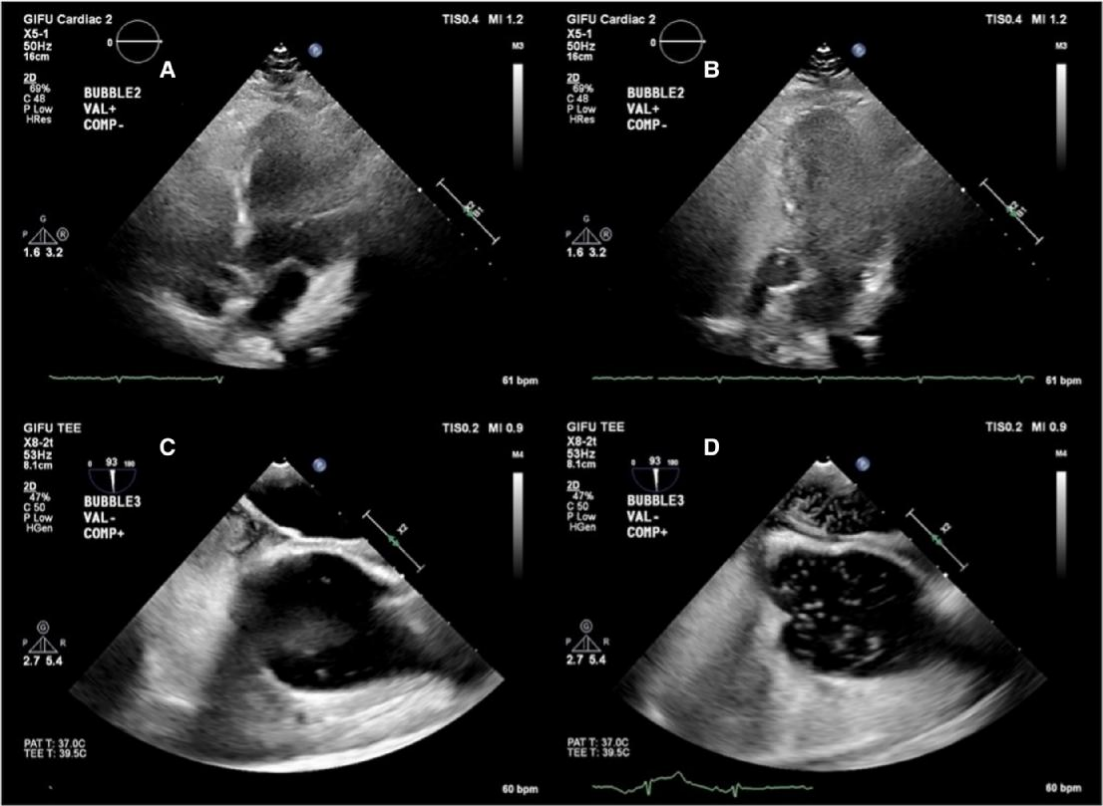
Bubble study before PFO closure. (*A*, *B*) Transthoracic echocardiography immediately after (*A*) and 3 cycles after (*B*) bubble injection. (*C*, *D*) Transoesophageal echocardiography immediately after (*C*) and 3 cycles after (*D*) bubble injection.
